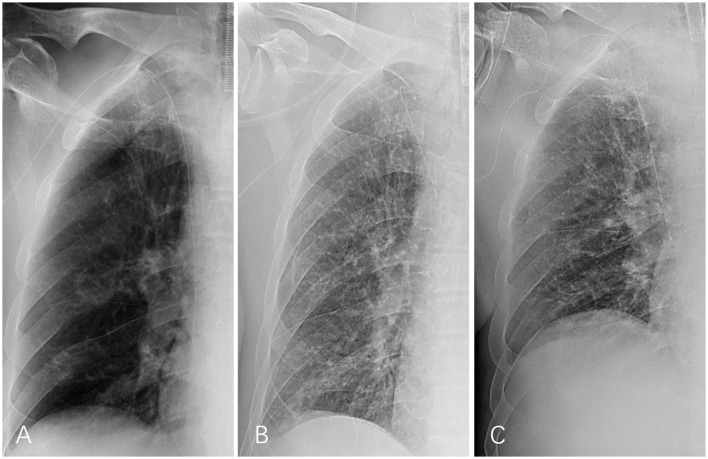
Serial chest X-ray findings during the therapy. (A) The PICC line was placed and ran straight along the vessel, with the tip correctly positioned at the junction of the superior vena cava and right atrium. (B) The proximal PICC segment remained tortuous on the chest X-ray obtained on April 12. (C) The proximal PICC segment returned to its normal shape on April 18.
